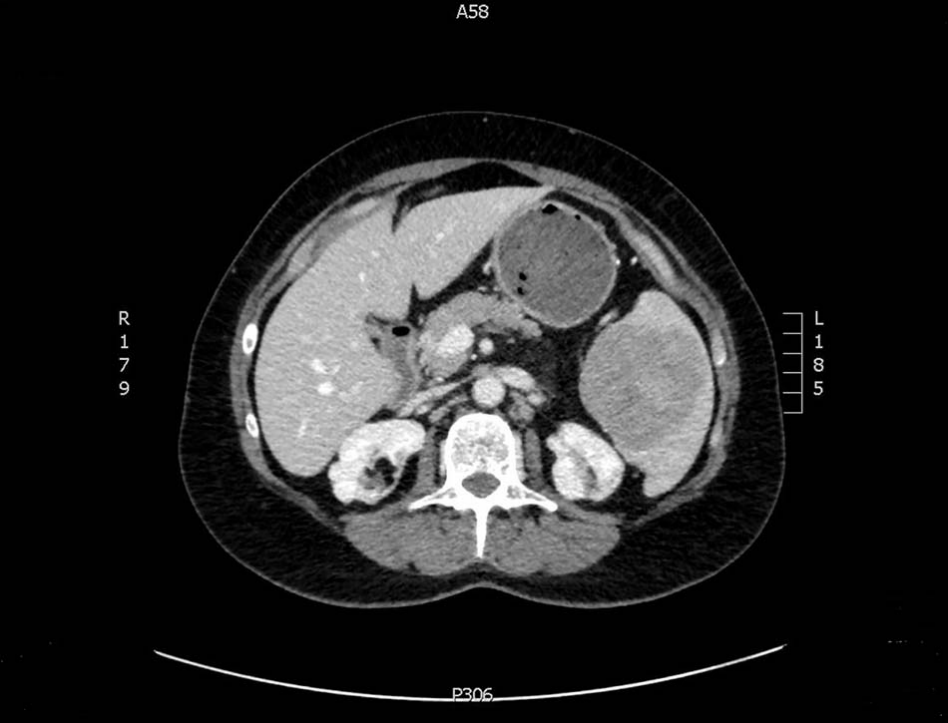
CT image of a splenic mass
